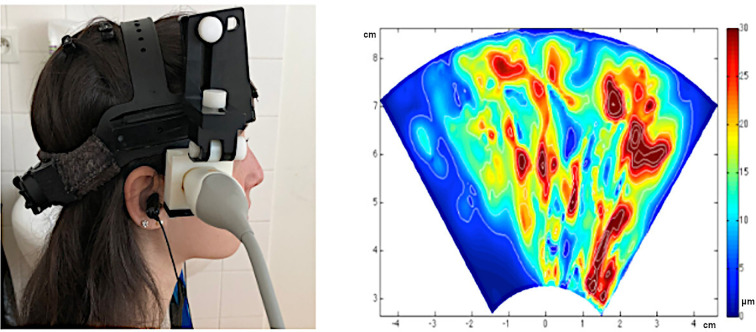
The typical set-up of the TPI equipment on a participant, with measurements taken from the right temporal position (left). The colour map shows the amplitude of brain tissue motion in the direction of the ultrasound beam with the brain moving inward (towards the midline) in systole (right). The map covers an axial slice of the brain centred on the Willis polygon (*x*-axis: posterior–anterior, *y*-axis: lateral–medial). The set-up shown in the figure was used in the M-Pulse study reported in [154].
